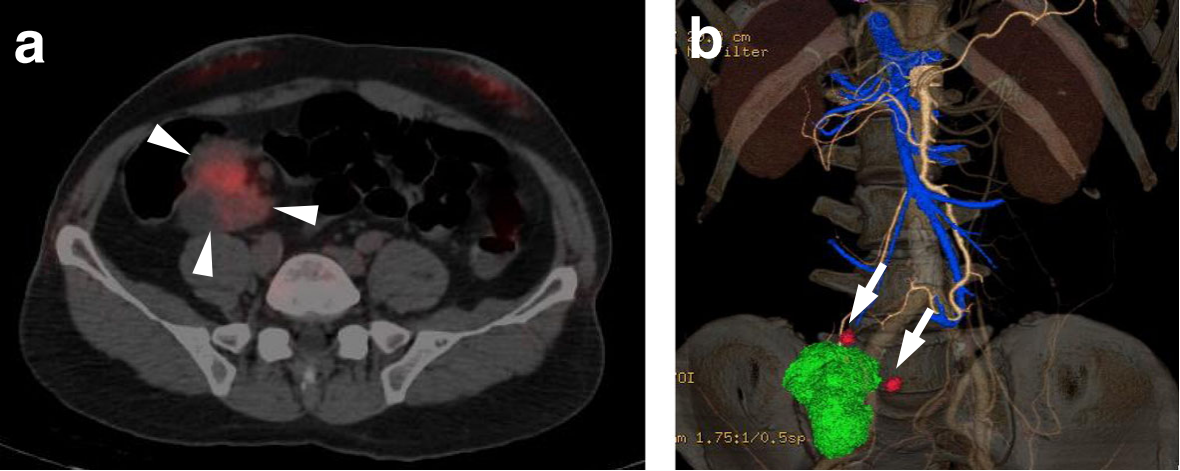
PET/CT colonography findings. a PET/CT showed polycystic tumor of 30 mm (*arrowhead*) with abnormal uptake on FDG-PET. b Lymph nodes neighboring the tumor were swelling (*arrow*)
